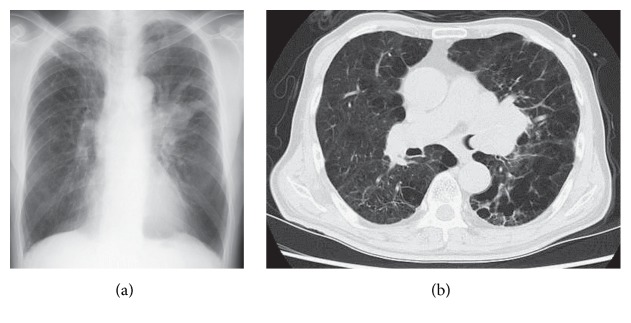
(a) Chest X-ray on admission revealed a mass in the left hilar region. (b) Chest computed tomography on admission showed a mass invading the left main bronchus.
